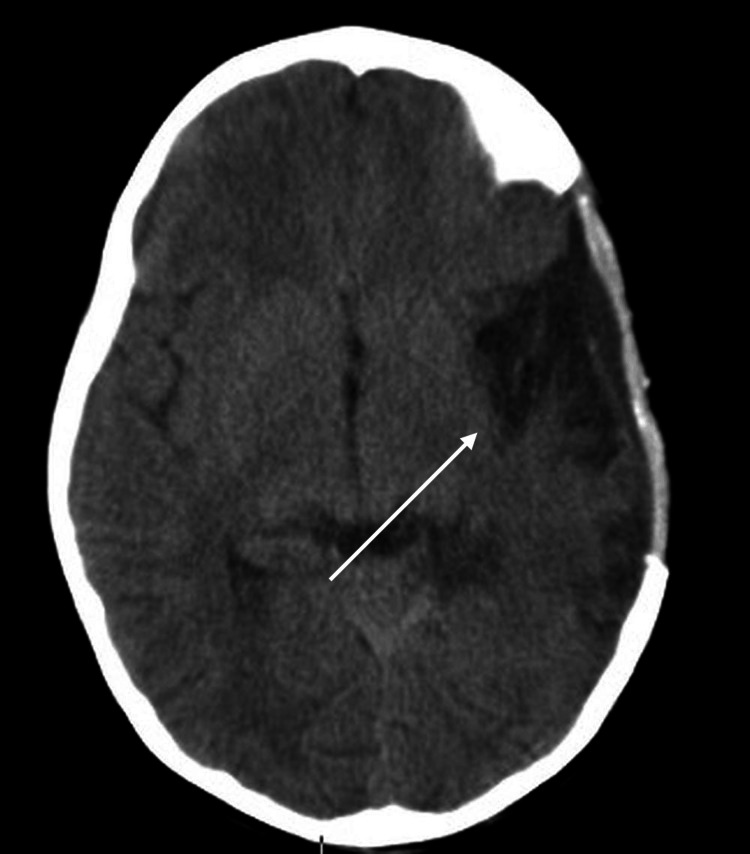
Postoperative CT at five months showed a stable craniectomy site with successful bone flap reinsertion and minimal residual encephalopathic changes (indicated by the white arrow).
